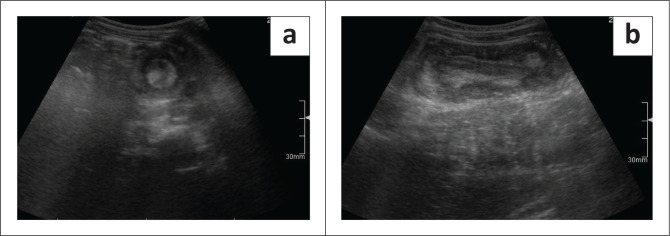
(a) The doughnut or bull’s eye or target sign is a pathognomonic transverse-view ultrasound finding and, in the clinical suspicion of intussusception, is highly sensitive and specific. (b) The pseudo kidney sign describes the appearance on the longitudinal ultrasound view of an intussusception.
